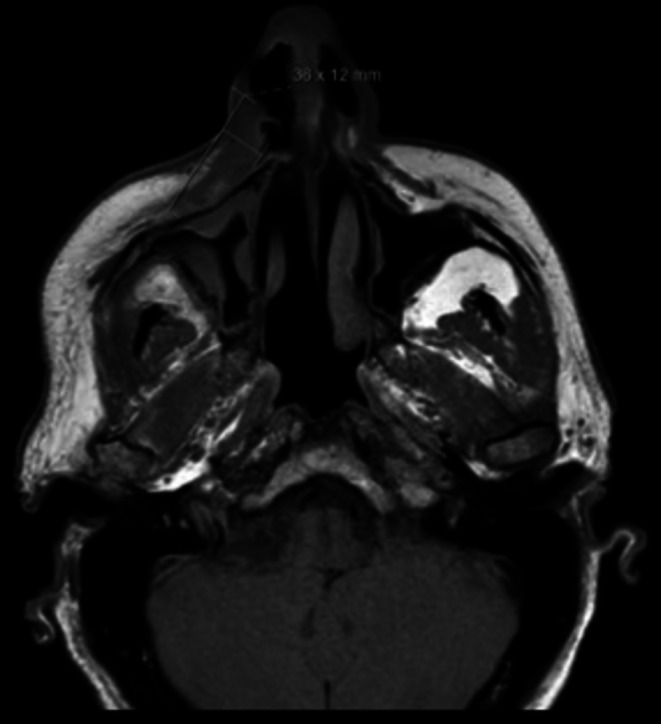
ENKTL of the nasal region. Axial T1‐weighted magnetic resonance imaging (MRI).
